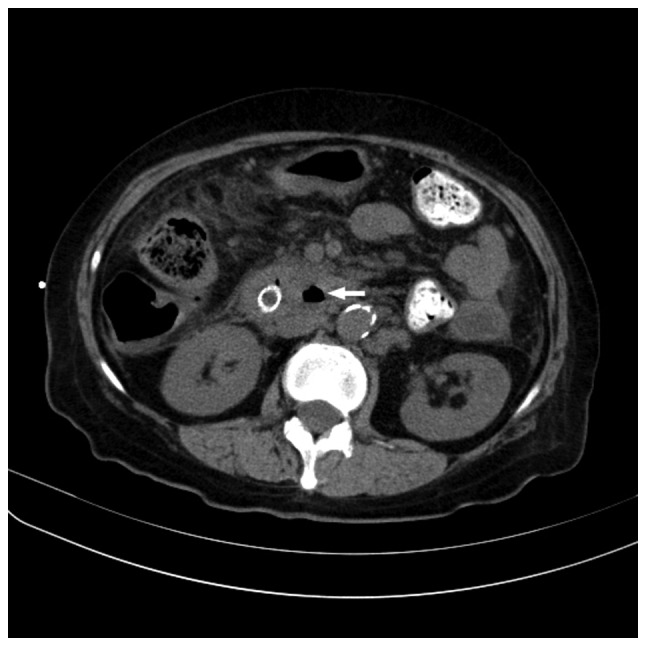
CT performed 4 days after PTBS revealing a tumor surrounding the intradiverticular papilla (white arrow) and the bile duct stent. PTBS, percutaneous transhepatic biliary stenting.
